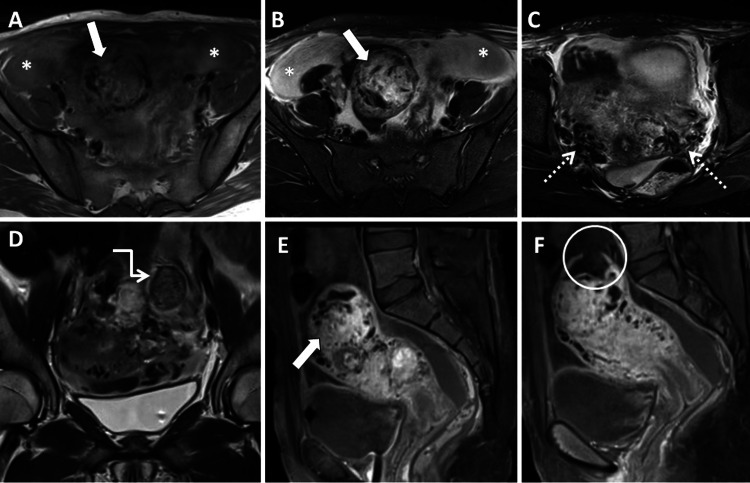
MRI of the pelvis with and without contrast. (A) T1-weighted axial, (B and C) T2-weighted fat-saturated axial, (D) T2-weighted coronal, and (E and F) sagittal T1 post-contrast images demonstrate a lesion in the fundus of the uterus that is T1 iso- to mildly hyperintense and T2 heterogeneously hyperintense. This lesion involves the myometrium and the superior-most portion of the endometrium (solid white arrows in A and B). Multiple areas of flow voids are seen within the lesion, adjacent to the lesion, in the remaining uterine myometrium, in the parametrium, and in the parauterine region of the pelvis (open white arrows in C). Also noteworthy is the enlarged left external iliac lymph node (white elbow arrow in D) and the hemoperitoneum (white asterisk in A and B). Post-contrast MRI images show intense, heterogeneous post-contrast enhancement of the lesion (solid white arrow in E) along with irregularity in the uterine outline and a discontinuity of the fundal myometrium due to uterine perforation (open white circle in F).
